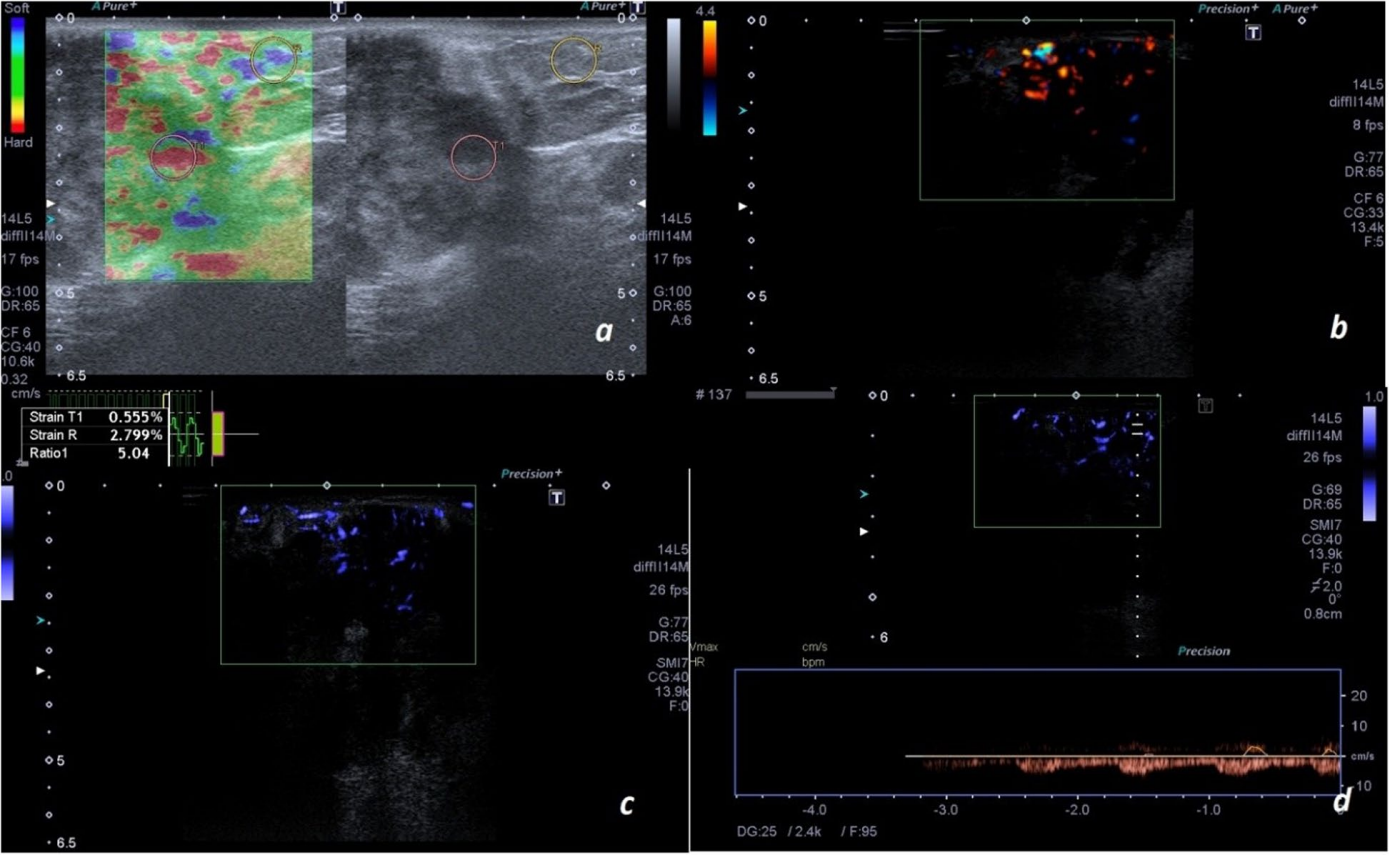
Pathologically proven invasive ductal carcinoma. a STE shows a high strain ratio. Both DUS (b) and SMI (c) show multiple dot-like and linear vessels within the mass. However, SMI shows a higher number of vessels within the lesion in comparison to DUS. Especially, SMI is superior in showing the vessels of the deeper portion of the mass. d There was arterialized flow on the spectral Doppler examination
